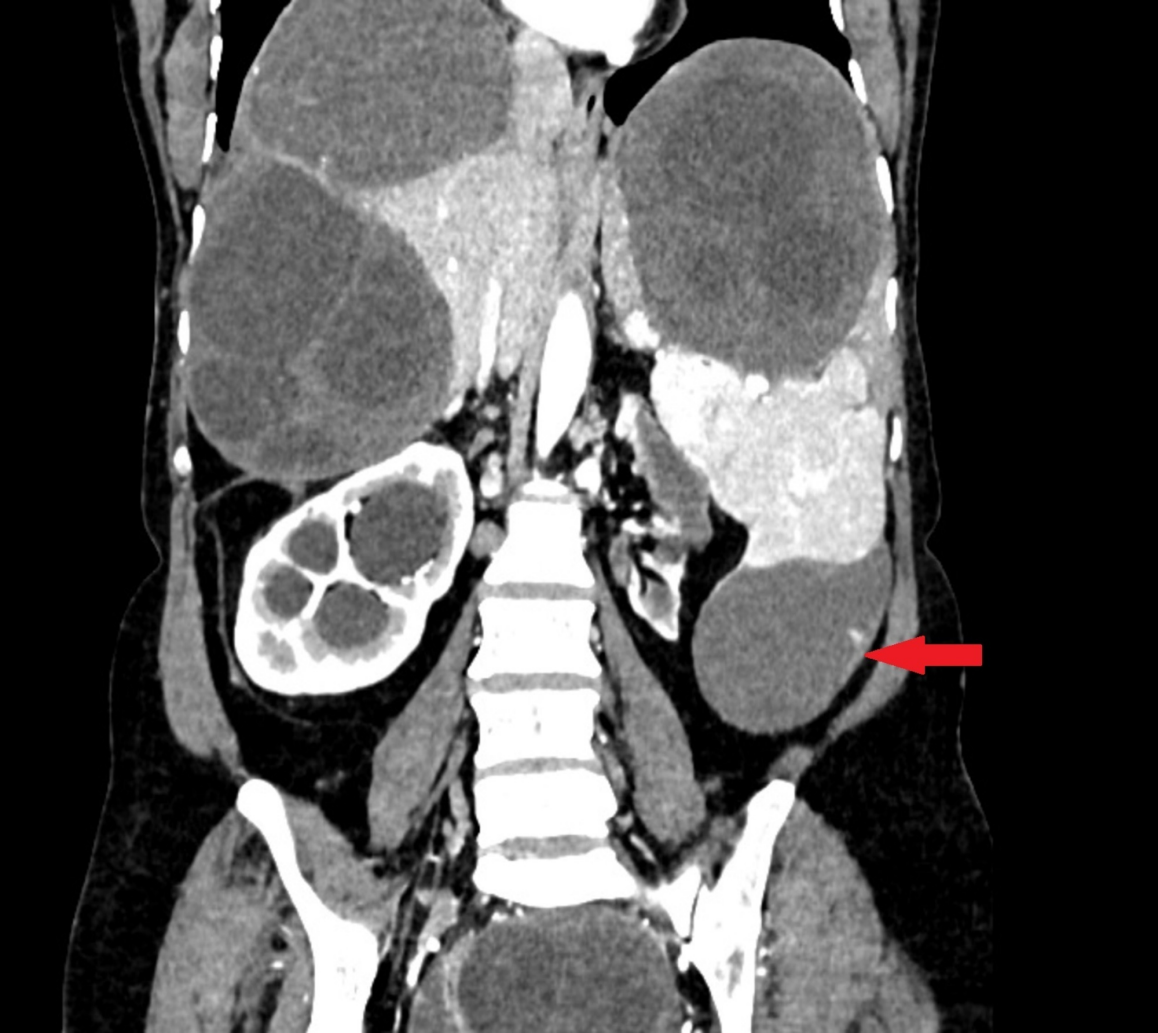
Splenic hydatid cyst on CT CECT abdomen coronal image shows a moderate-sized, well-defined, rounded cystic lesion in the spleen (red arrow) with septation. CECT: contrast-enhanced computed tomography, CT: computed tomography
